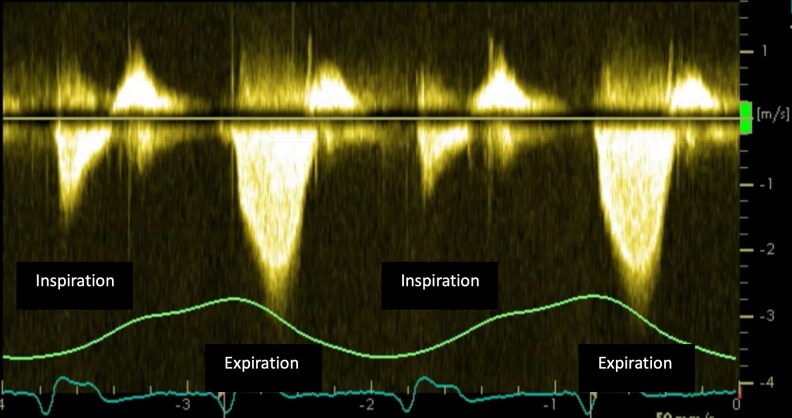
Impact of respiration on Doppler velocities in functional tricuspid regurgitation. During each inspiration, the CWD TR signal velocity profoundly decreases and adopts a v-waveform due to increased EROA during inspiration, whereas during expiration, the CWD signal becomes less triangular with higher TR velocities because EROA decreases.
